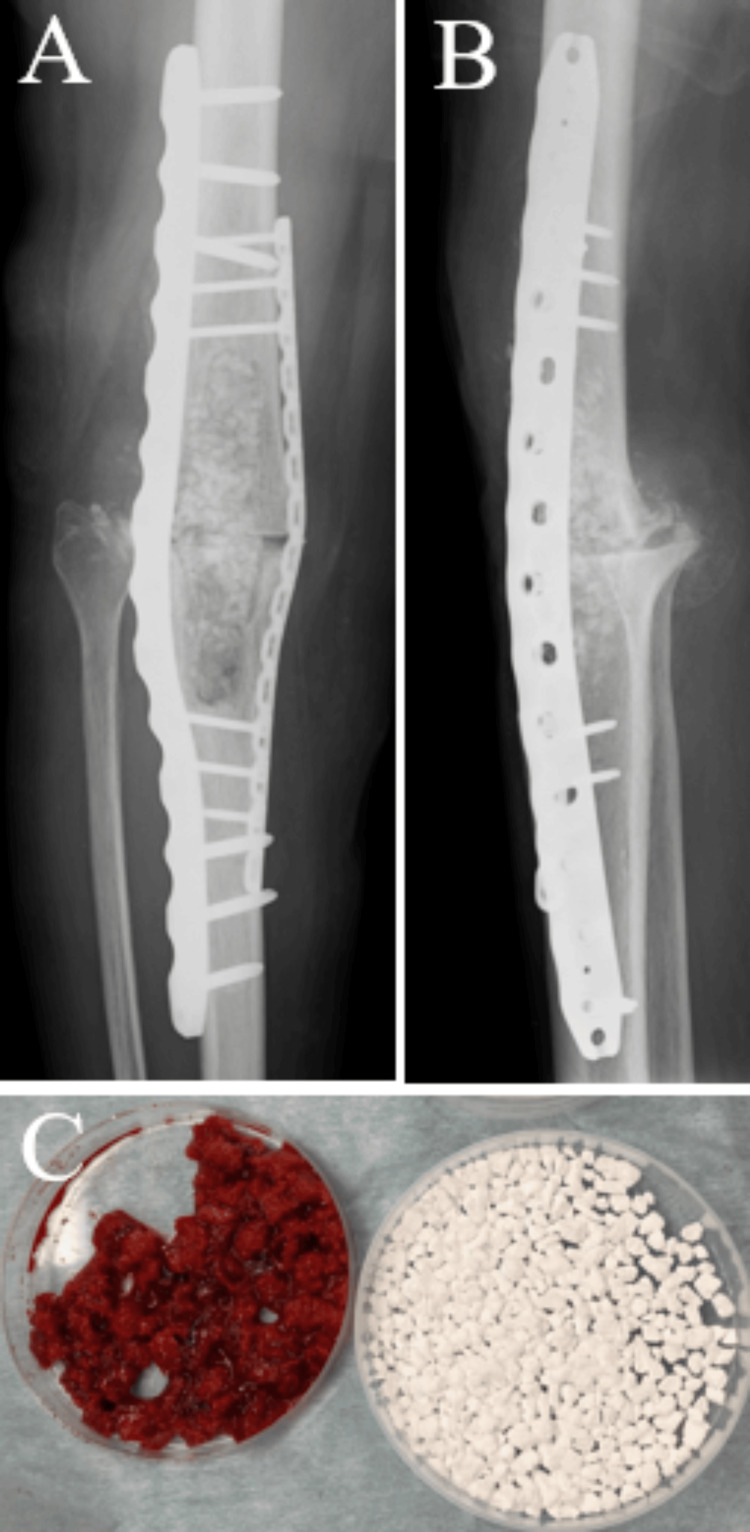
At the second stage, bone grafting was performed. (A) Anteroposterior radiograph of the knee joint. (B) Lateral radiograph of the knee joint. (C) Grafted with the same amount of beta-tricalcium phosphate (β-TCP) mixed with cancellous bone taken from the iliac bone.
